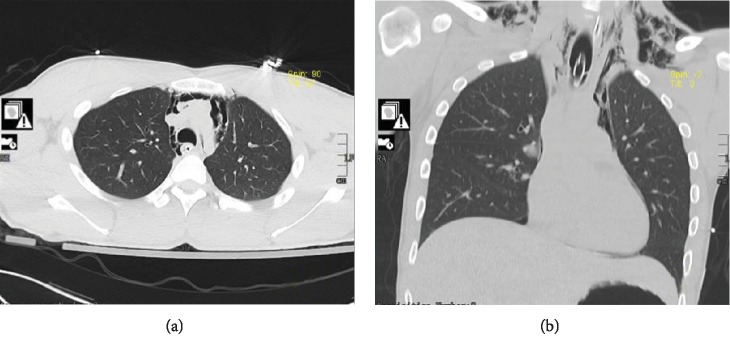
(a) Chest computed tomography (CT) axial view showing extensive pneumomediastinum with air dissecting to the peribronchovascular interstitial sheaths and visceral pleura. (b) Chest computed tomography (CT) coronal view showing extension of the subcutaneous emphysema on the supraclavicular area.
